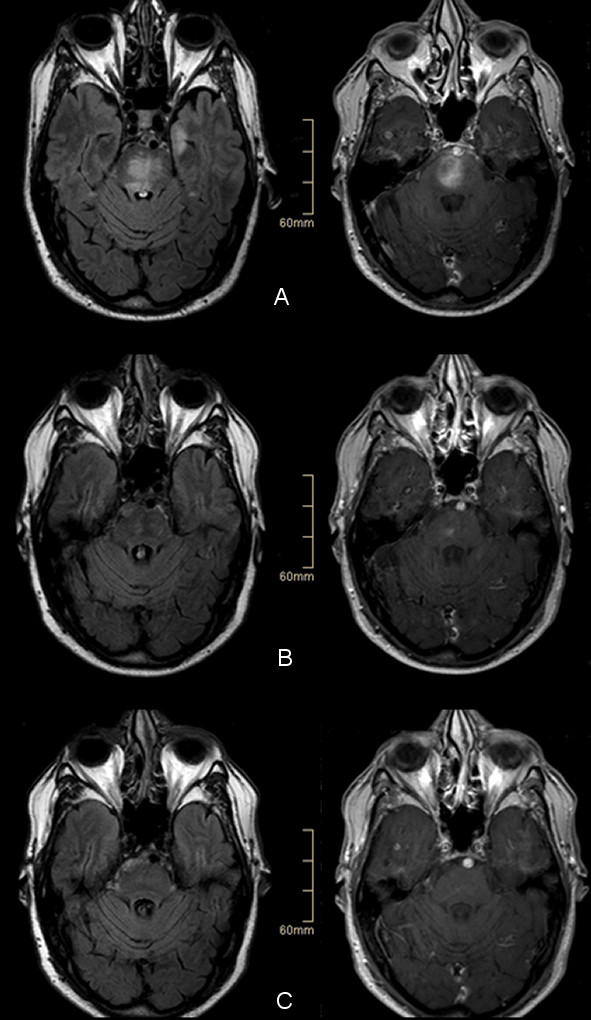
MR imaging prior to and after HD-MTX-based chemotherapy (left column FLAIR, right column: contrast enhanced T1-weighted imaging)MR imaging prior to therapy (A) and at follow-up imaging at the end of 6 courses of chemotherapy with a strong reduction of contrast-enhancing lesions (B). Nineteen months after initiation of treatment MR imaging showed complete regression of marked FLAIR hyperintensities and contrast enhancement in the brain stem (C).
